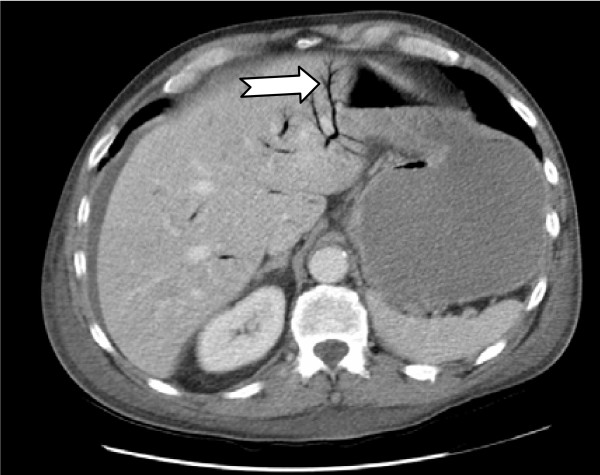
CT scan showing aerobilia (arrow) consistent with a cholecysto-enteric fistula and free fluid within the abdomen.
